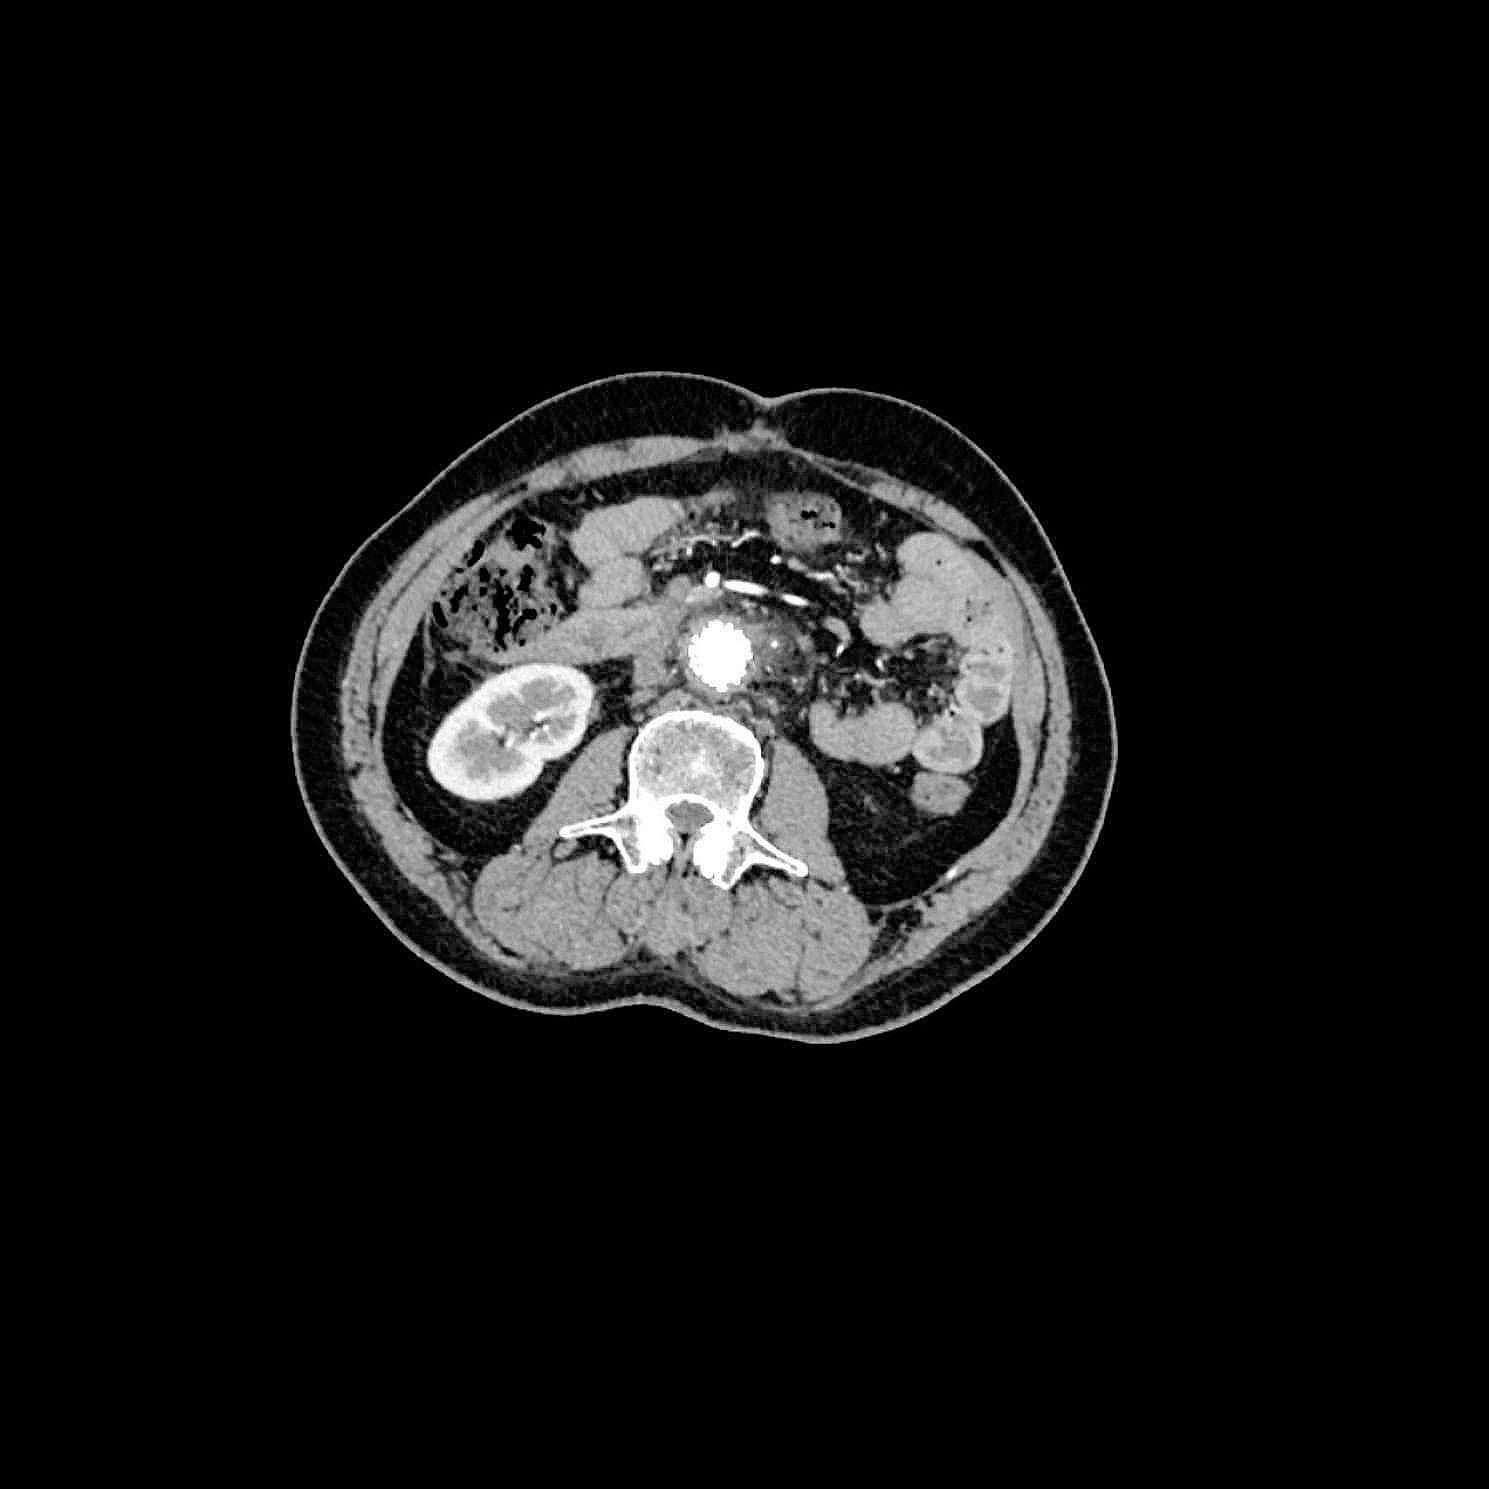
The CTA performed half a year after the operation
